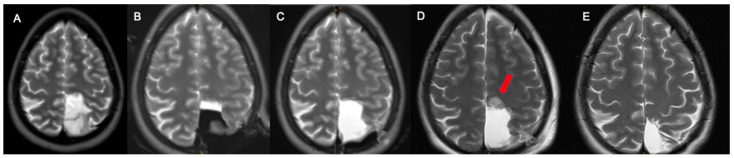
(A) shows T2 hyperintense lesion. (B) depicts intraoperative MRI and gross total resection of tumor confirmed in ultra-early MRI (C). (D) shows early postoperative MRI and surgical induced artefacts mimicking (red arrow) residual tumor. (E) late follow up MRI confirmed gross total resection.
